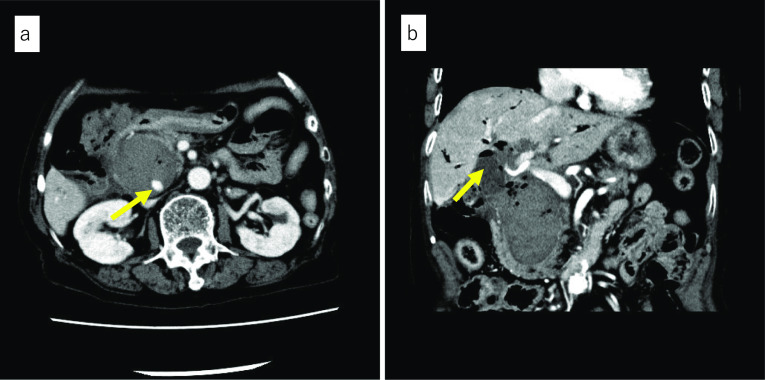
Preoperative enhanced CT findings. a Transverse image. Contents of the cystic lesion of the pancreatic head reveal a relatively high CT number. An arrow shows pseudoaneurysm in the cystic lesion. b Coronal image. The gallbladder had been resected. An arrow shows the suspicious structure of the elevated jejunum adjacent to the gallbladder bed
